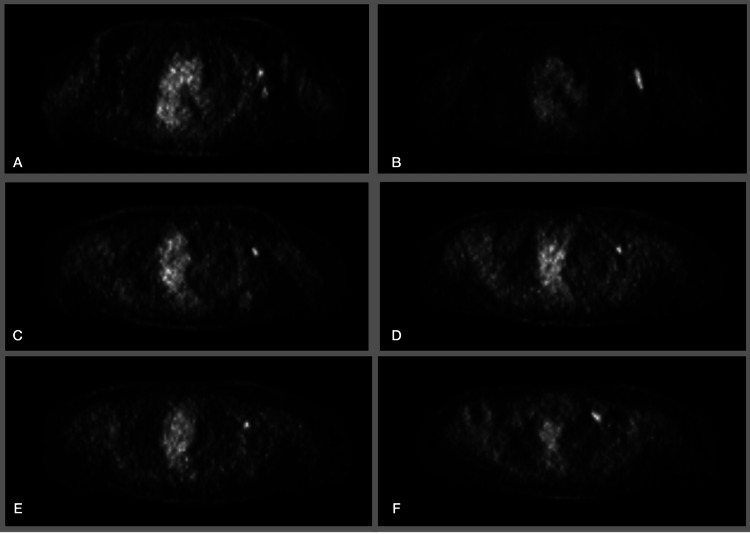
Positron emission tomography-computed tomography of the chest. A-E: Increased uptake in left axillary lymph nodes. F: Increased uptake in left supraclavicular lymph nodes.
